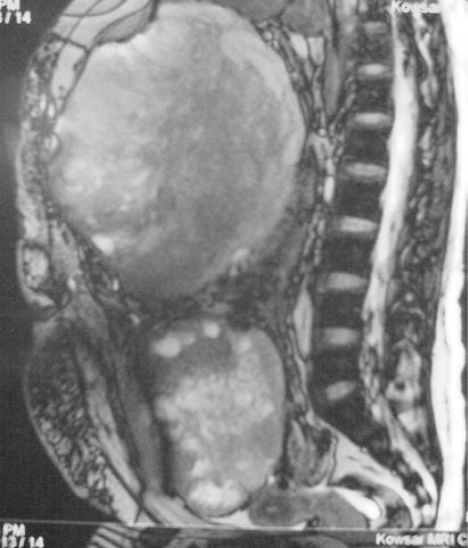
Magnetic Resonance Imaging shows huge heterogeneously enhancing mass with some necrotic foci which has filled the abdominal and pelvic cavities.
